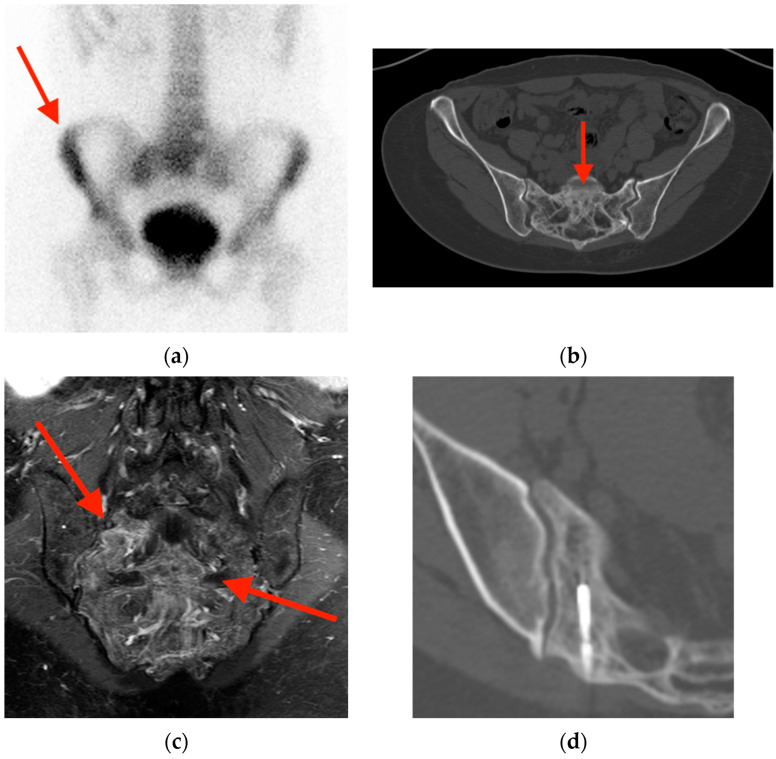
(a) Bone scintigraphy in a 54-year-old woman with elevated alkaline phosphatase reveals heterogeneously increased uptake in the sacrum, most prominent at the right sacral ala (red arrow). (b) CT shows diffuse cortical and trabecular thickening with mild bone expansion (red arrow). (c) Post-gadolinium fat-suppressed coronal T1-weighted MRI shows diffuse bone enhancement with associated coarse trabecular thickening (red arrows). (d) During the course of work-up, the sacrum was biopsied and pathology revealed active Paget’s disease. CT = computed tomography. MRI = magnetic resonance imaging.
